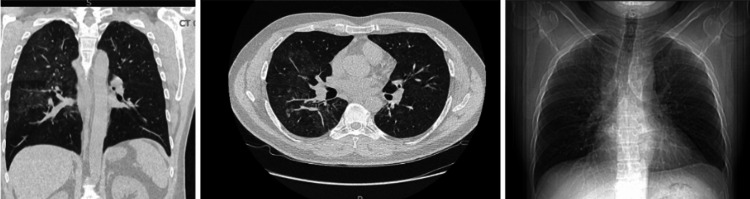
High-resolution CT and chest X-ray demonstrate ground-glass opacities and diffuse interstitial infiltrates in a patient with PCP. Courtesy of Leonard J. Chabert Medical Center. PCP: *Pneumocystis jirovecii* pneumonia.
